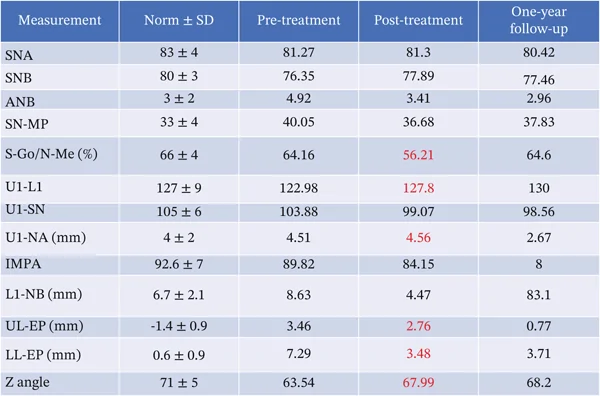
One‐year follow‐up radiographs: (A) panoramic radiograph; (B) lateral cephalogram; and (C) lateral cephalogram tracing.
